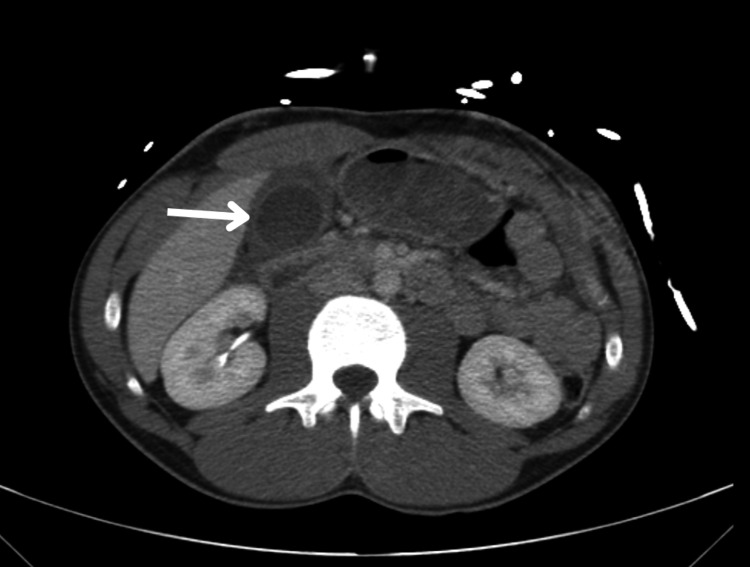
Axial computed tomography image demonstrating the distended gallbladder without calculi with circumferential wall thickening (arrow)
